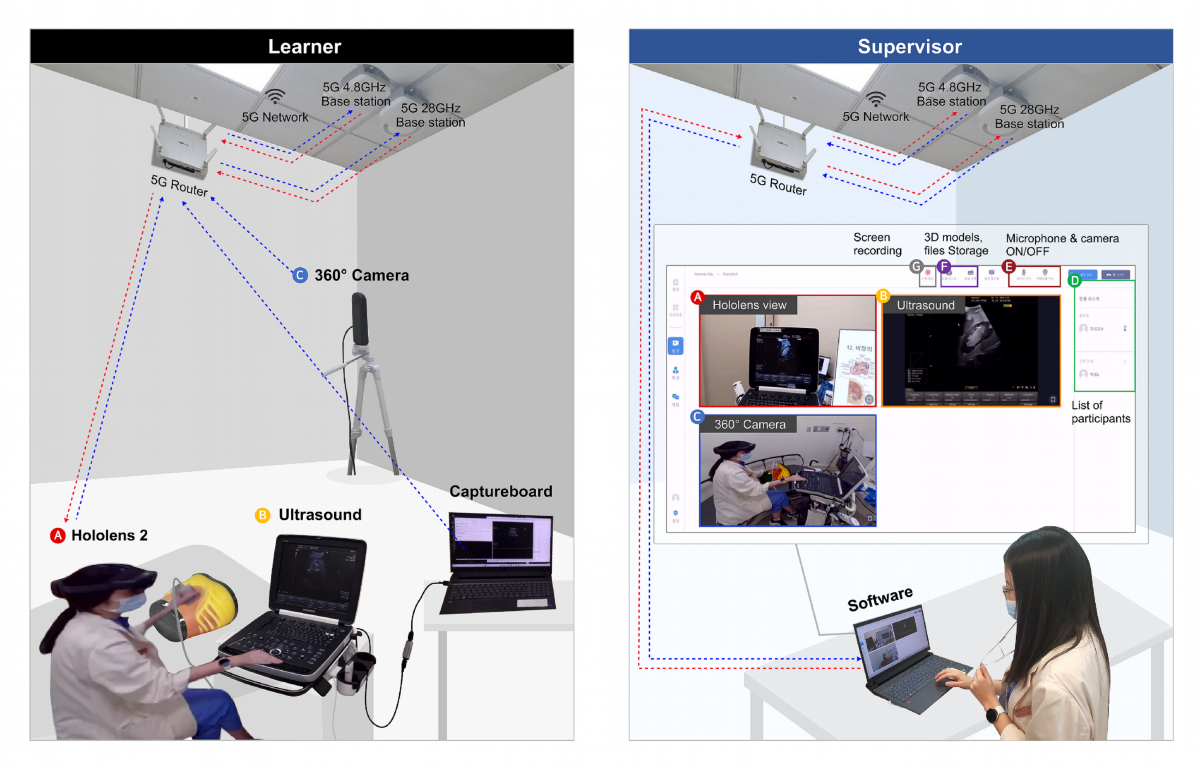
System architecture of MR telesupervised ultrasound education platform. The learner’s part consists of (A) an HMD, (B) an ultrasound device, and a laptop to capture ultrasound images through the capture board, and (C) a 360° camera. The software used by the supervisor displays the video streamed from (A), (B), and (C). Additionally, there is a (D) list of participants, (E) communication with the learner via microphone and camera that can be turned on and off by the supervisor, (F) the ability to send 3D objects, files, and images from the storage, and (G) a recording function. HMD: head-mounted display; MR: mixed reality.
